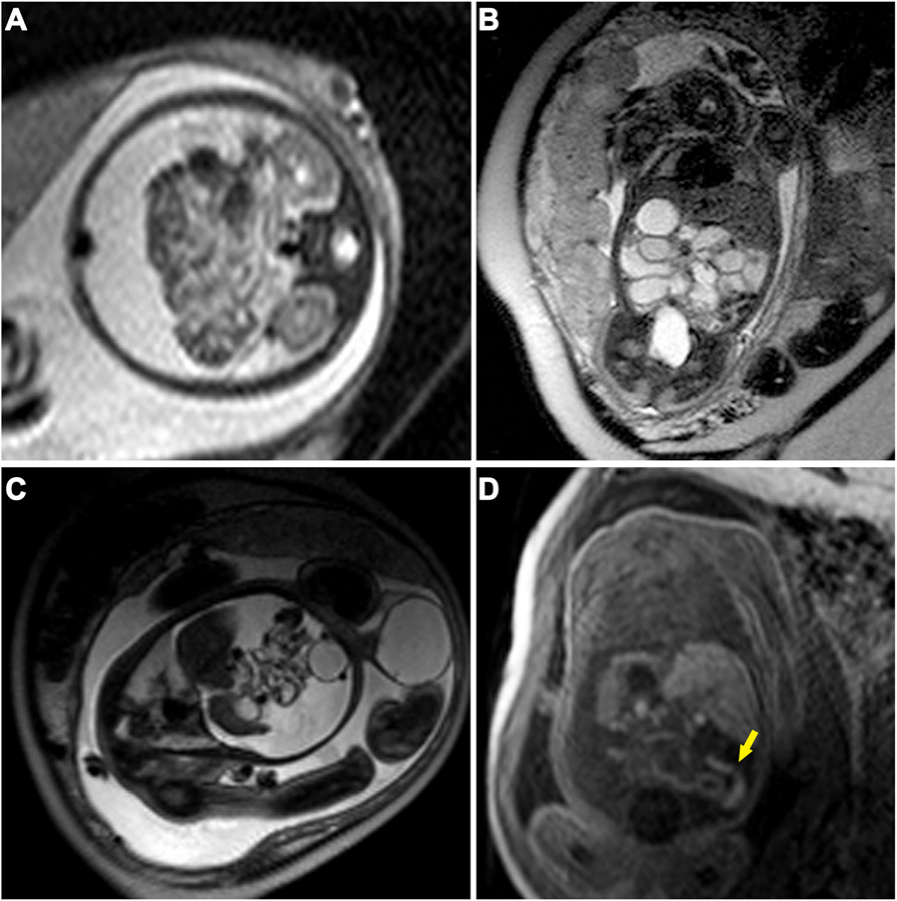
Fetal MRI findings of meconium peritonitis in two fetuses receiving surgery. a-b A 30-week-old fetus with MP. A transverse 1.5-T T2-weighted image (TR = 15,000 ms, TE = 120 ms) indicates a large amount of ascites and gathering bowel loops (a) and dilated bowels (b). C-D. A 35-week-old fetus with MP. A sagittal 1.5-T T2-weighted image (c, TR = 15,000 ms, TE = 120 ms) reveals a high amount of free ascites in the peritoneal cavity, gathering and distortion of poor filled small intestines, and significant swelling in the left scrotum. A coronal T1-weighted image (d, TR = 234 ms, TE = 4.6 ms) shows distal micro-colorectum (yellow arrow)
